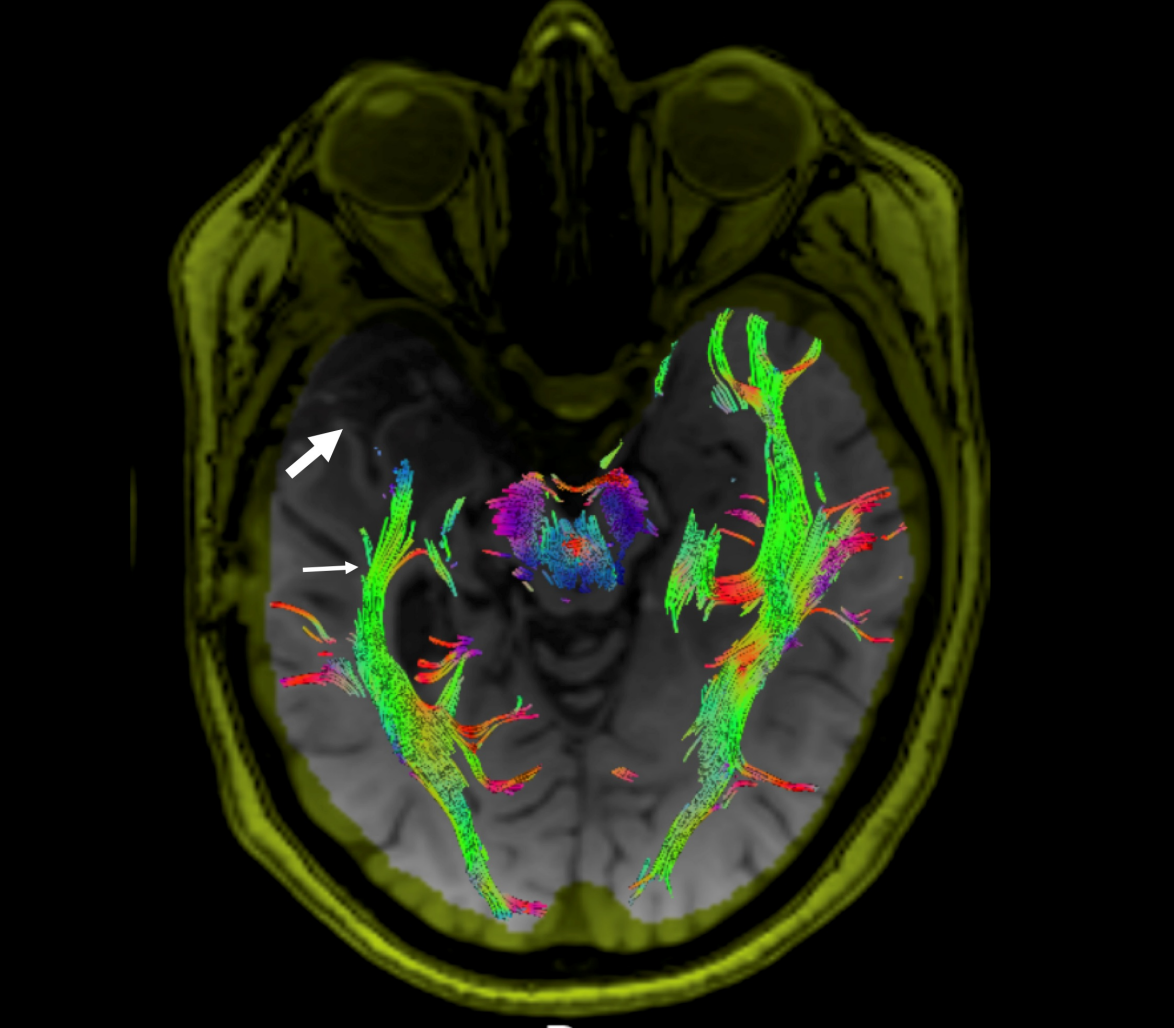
Diffuser tensor imaging and tractography of the optic radiations in a case of unilateral mesial temporal sclerosis (MTS) following resection of the right anterior temporal lobe. The optic radiations are depicted by using tractography. The tracts are overlaid on T1-weighted images. Postoperative changes, including encephalomalacia, are demonstrated within the right middle cranial fossa and the surgical site is denoted by the large arrow. The right optic radiations are thinned and disrupted on the ipsilateral side (small arrow).
